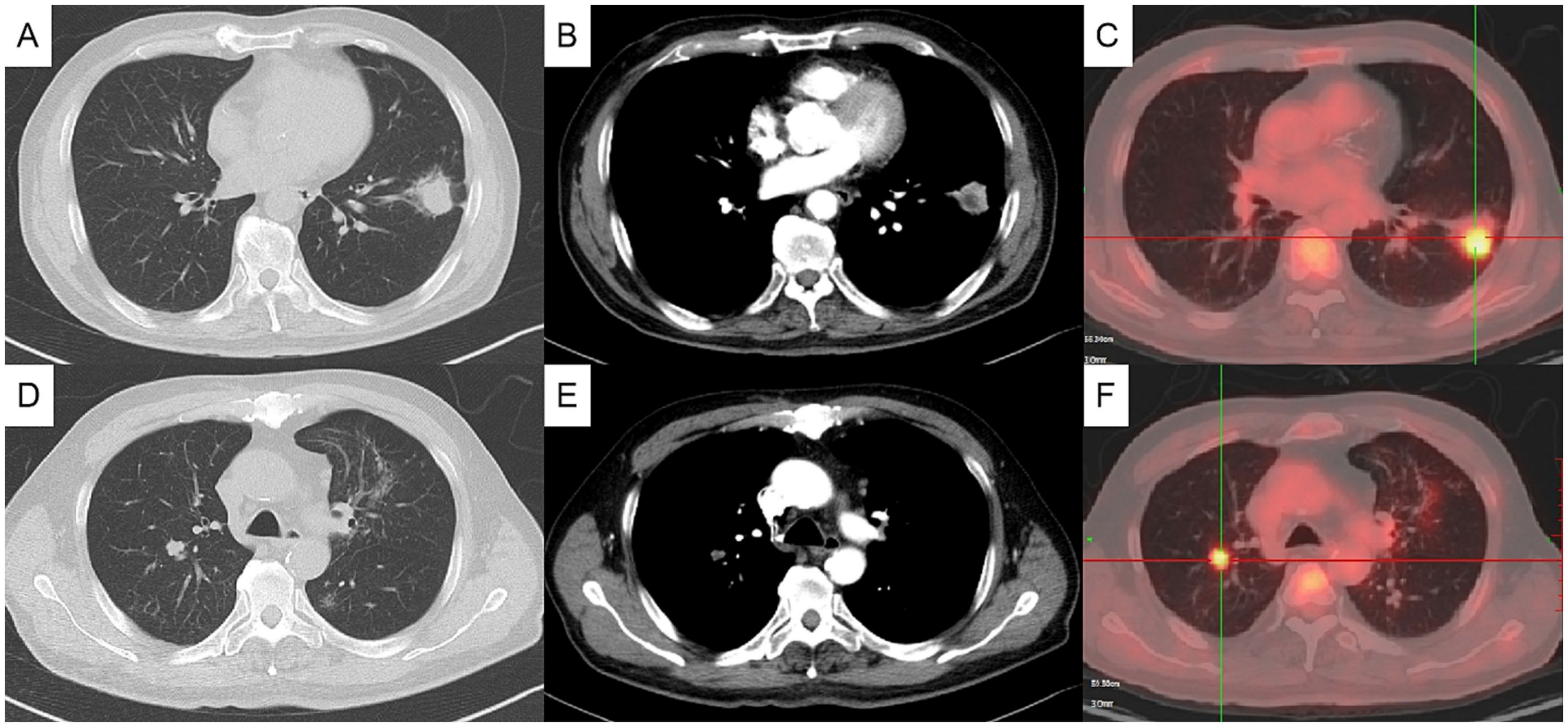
Initial chest computed tomography (CT) and positron emission tomography/computed tomography (PET/CT) (axial). Chest CT showing a mass in the anterior and inner basal segment of the left lower lobe, about 3.1×2.5 cm, with signs of lobulation, burr, pleural adhesion and local bronchial truncation (A,B). Chest CT showed multiple small patch shadows around the bronchi of the right upper lobe and small solid nodule in the posterior segment of the right upper lobe, appropriate 1 cm in size (D,E). PET/CT indicating hypermetabolism in the nodules of the left lower lung (C) and the right upper lung (F), with SUVmax value of 5.7 and 4.7, respectively. (A, C pulmonary window, B, D mediastinal window).
